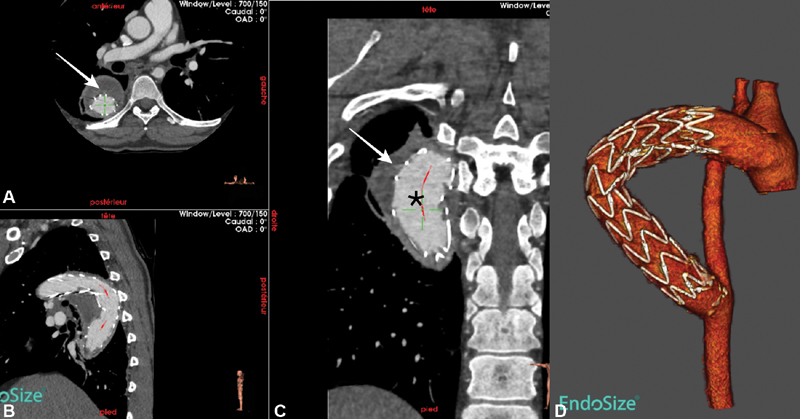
Postoperative computed tomography scan findings with three-dimensional (3D) reconstruction, confirming false aneurysm exclusion and thrombosis, correct positioning of the stent-graft, and the absence of endoleak. (
